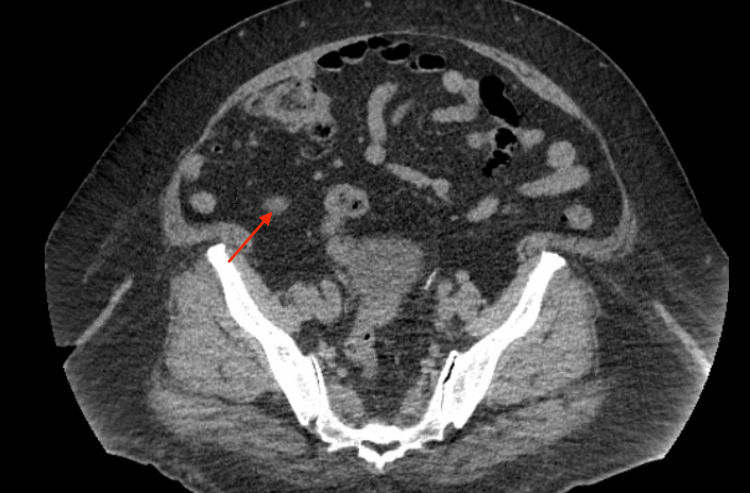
Second image of the patient’s initial CT scan of the abdomen and pelvis, showing evidence of mesenteric and retroperitoneal lymphadenopathy. CT, computed tomography
